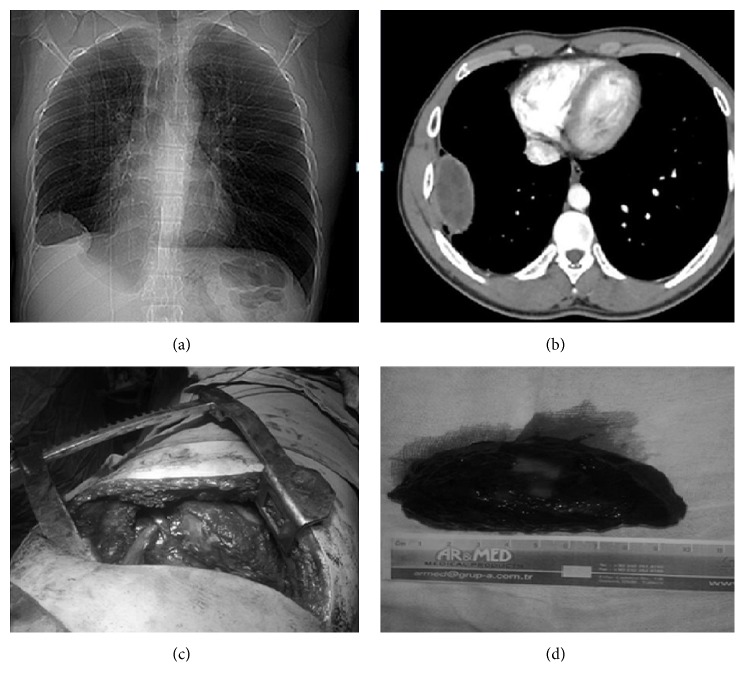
Chest X-ray image of the extrapulmonary intrathoracic hydatid cyst in the first case (a). Computed tomography image of the extrapulmonary intrathoracic hydatid cyst in the first case (b). Operation images of the first case (c). Image of the totally resected hydatid cyst (d).
